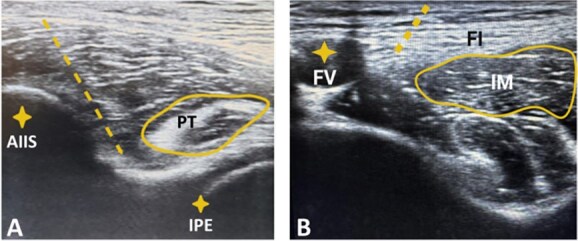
(A) Ultrasound image demonstrating the PENG block; anterior inferior iliac spine (AIIS), psoas tendon (PT), iliopectineal eminence (IPE), and dotted arrow represents needle direction. (B) Ultrasound image demonstrating the FICB; iliacus muscle (IM), fascia iliaca (FI), femoral vessels (FV), and dotted line represents needle direction.
